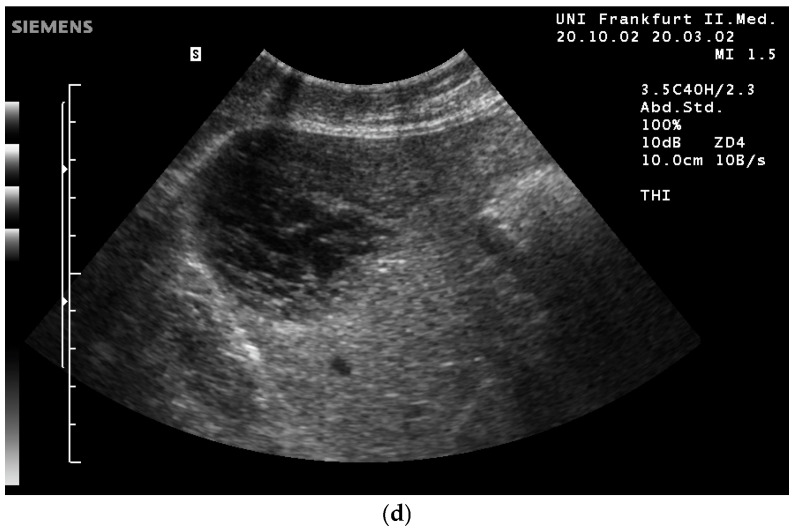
Examples of the comparison of colors using photopic in the mentioned study for experts and students. Artifact-free representation of a liver sarcoma at the same point in time using the four available photopic colors sepia (a), blue (b) and green (c) as well as gray (d). Saturation and intensity differ. Relevant factors influencing the photopic transformation of image information include image brightness, color type and color saturation. In order to realize a sonography in daylight, it is necessary to increase the image brightness and to increase the contrast as much as possible. All steps must be realized without artifacts.
